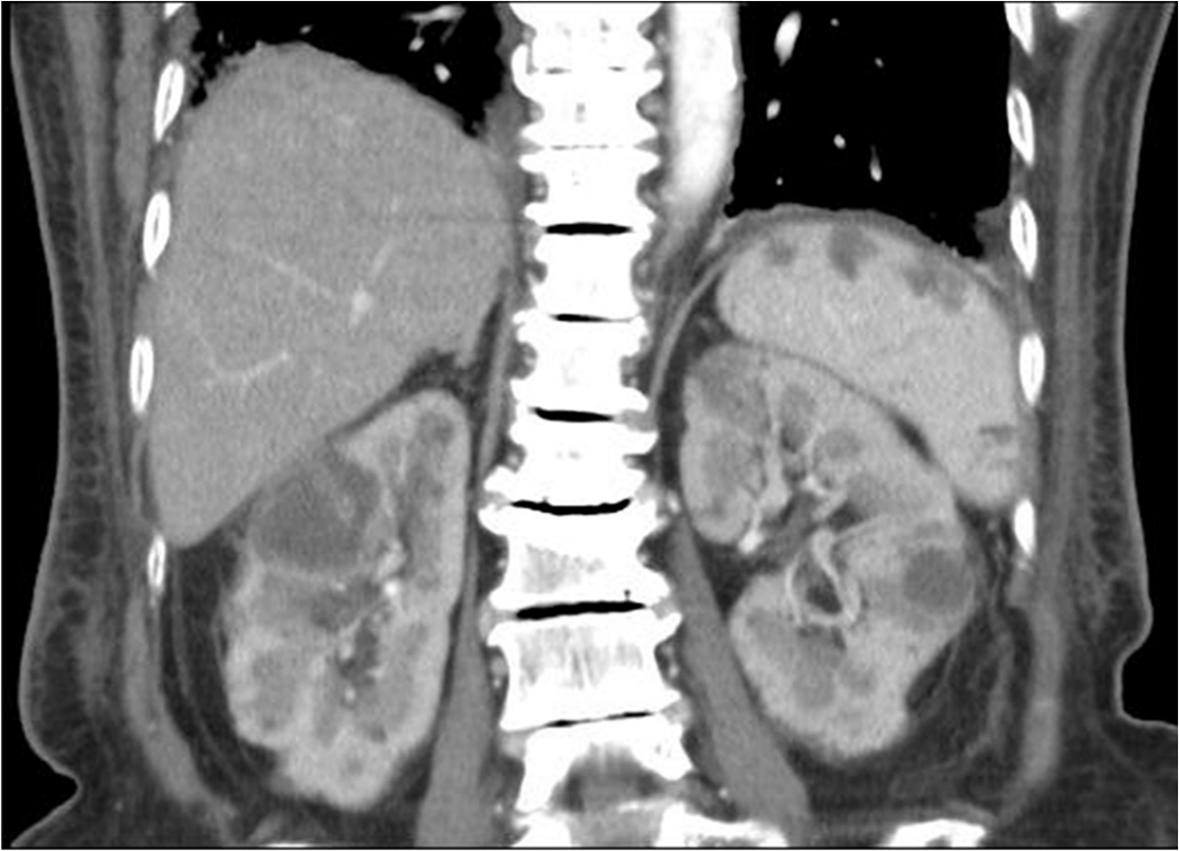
Computed tomography scans of the abdomen revealing several rim-enhancing hypodense lesions in bilateral kidneys and the spleen.
